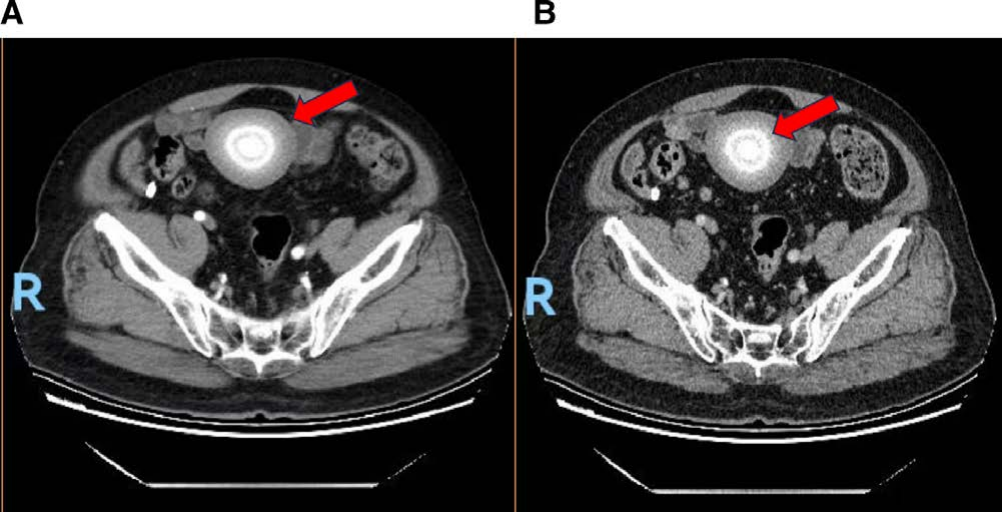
CT imaging of abdominal masses. (A) arterial phase; (B) venous phase. CT = computed tomography.
